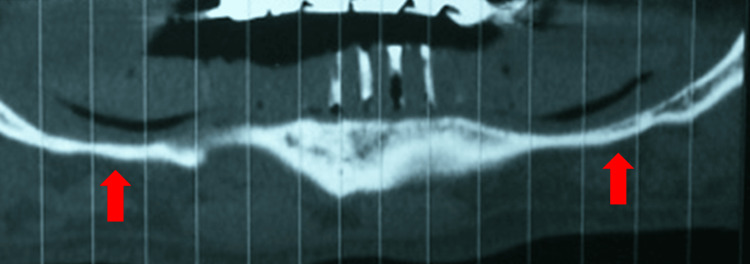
DentaScan with X-ray guide revealing a thin posterior ridge.
